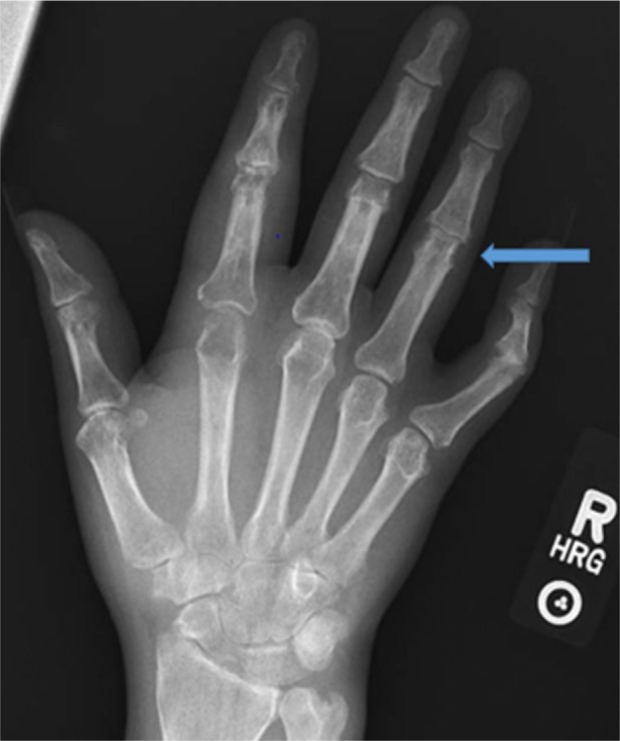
Hand X-ray suggesting early pencil cup deformity.
